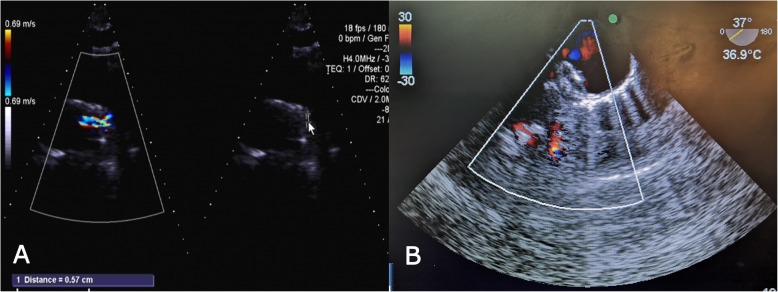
a Preoperative echocardiography shows patent ductus arteriosus. b Postoperative echocardiography shows that the occluder is in a good position with no residual shunt
